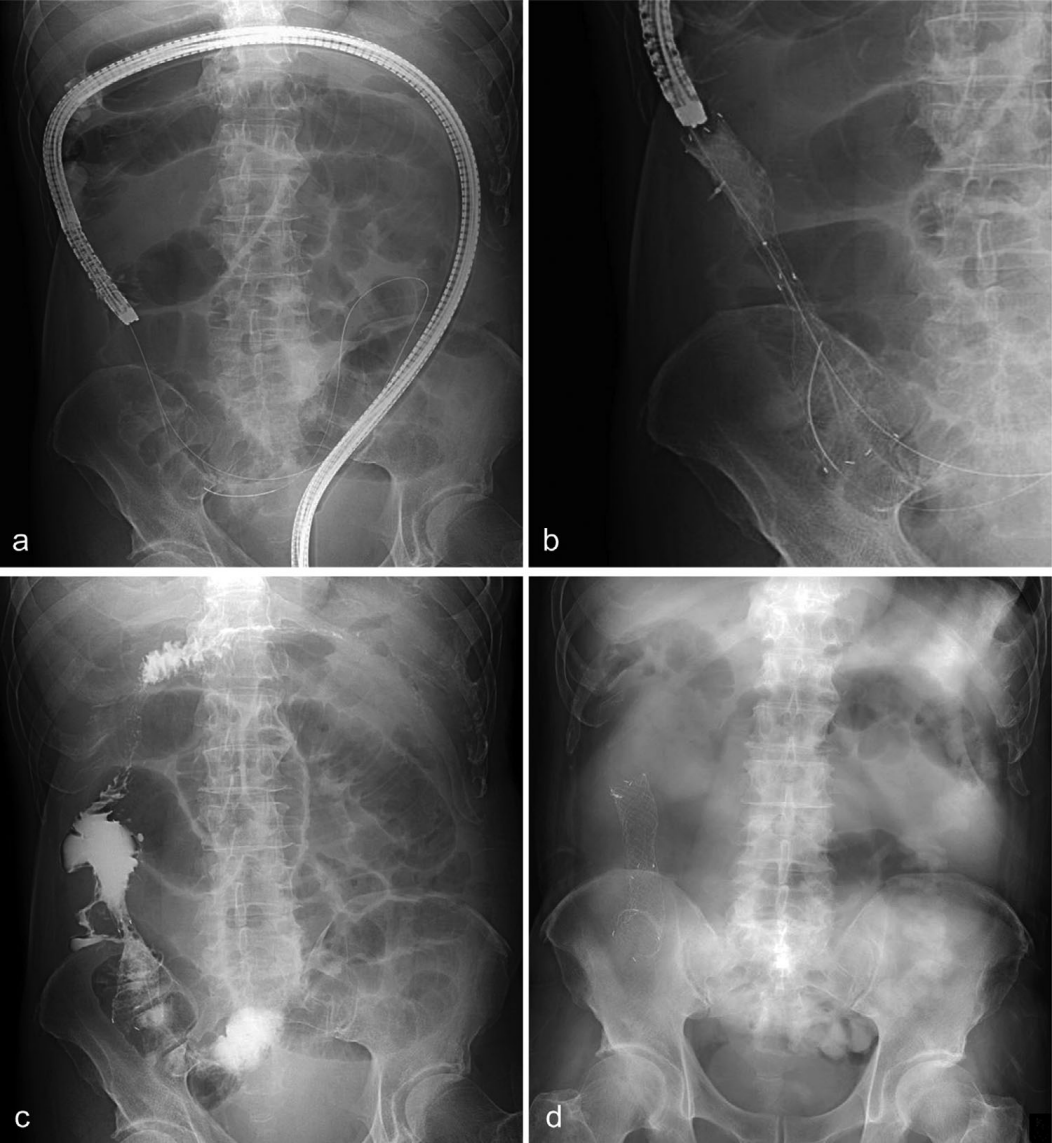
Colonic stenting across the ileocecal valve. (a) The PCF‐H290TI scope (Olympus, Tokyo, Japan) is advanced to the stenosis by following the guidewire. The guidewire is threaded through a pre‐catheter from the channel to the end of the scope. (b) A self‐expanding metallic stent (SEMS) is placed through the scope. (c) Fluoroscopic image after SEMS placement. (d) Abdominal radiograph taken the day after SEMS placement.
